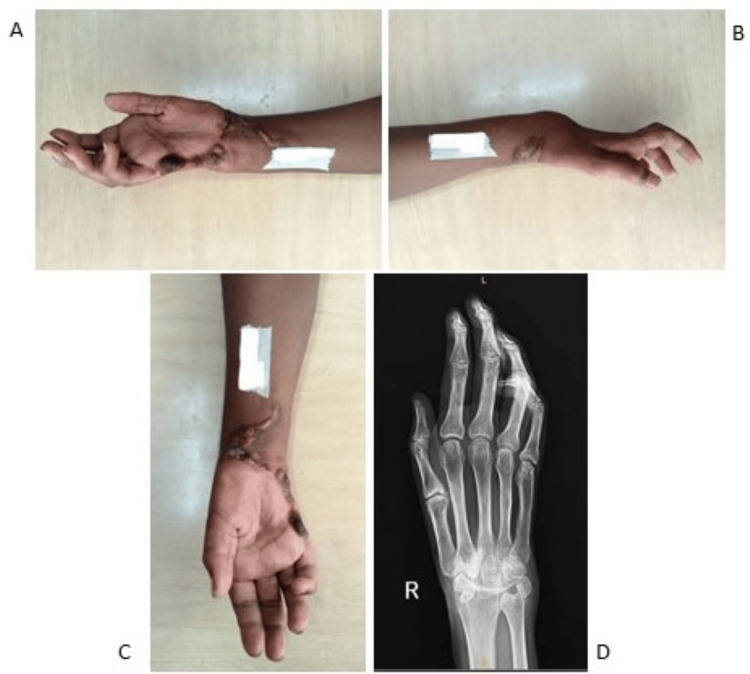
Corrected wrist deformity of patient 2 postoperatively (A). Decrease in the flexor contracture postoperatively (B). Improvement in the stiffness of MCP joint and improvement of the function of fingers postoperatively (C). Postoperative X-ray of the right wrist after carpectomy of patient 2 (D). MCP, metacarpophalangeal
